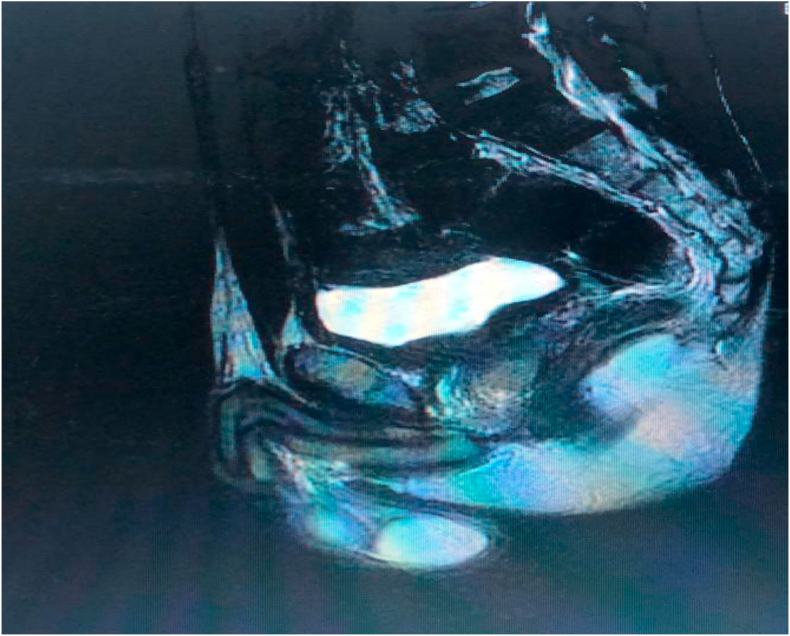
Pelvic Magnetic resonance imaging (MRI) showing a tumor melting and adenopathies disappearance.
